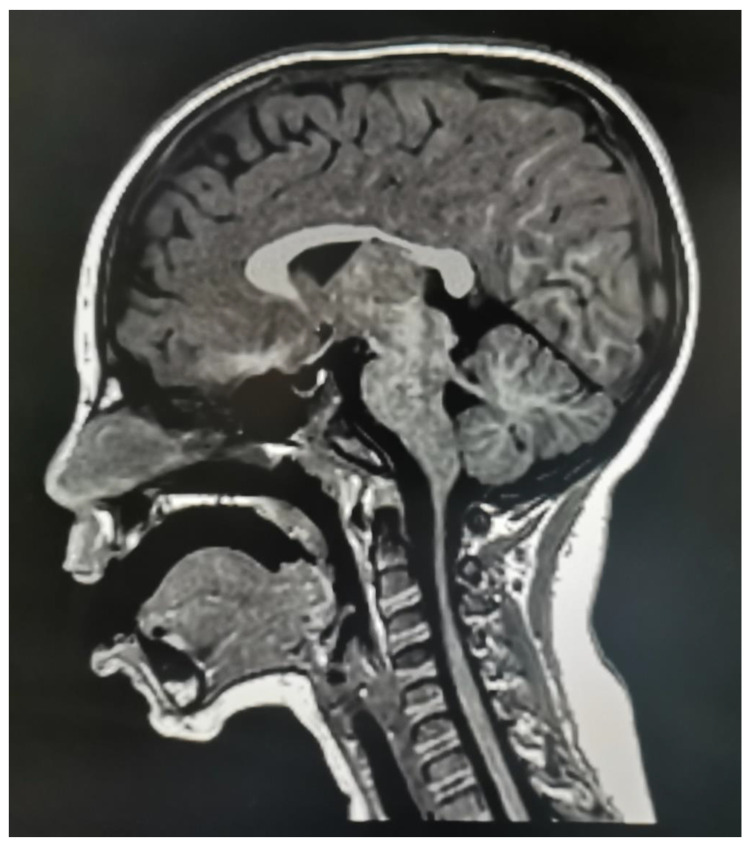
Cerebral magnetic resonance imaging (MRI) scan showing posterior hypoplasia of corpus callosum.
